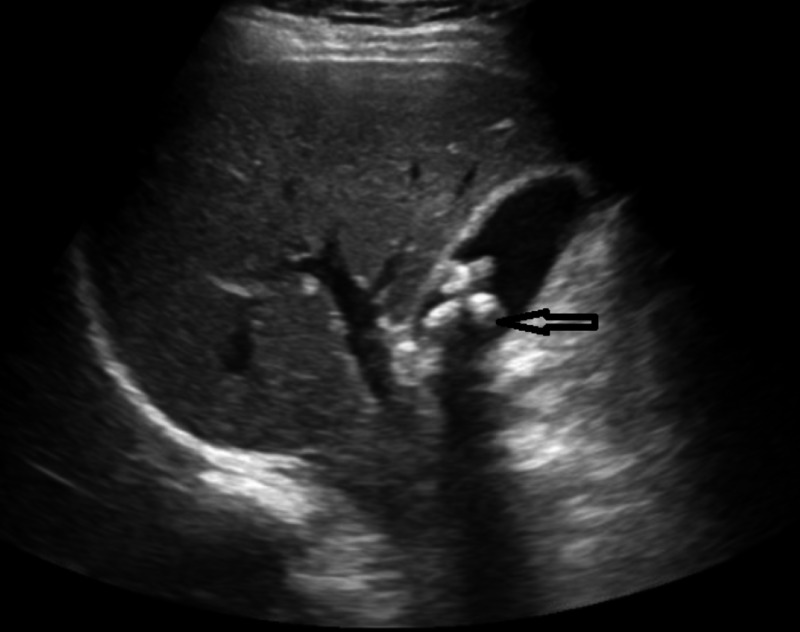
Ultrasound of liver Black arrow demonstrates cholelithiasis
